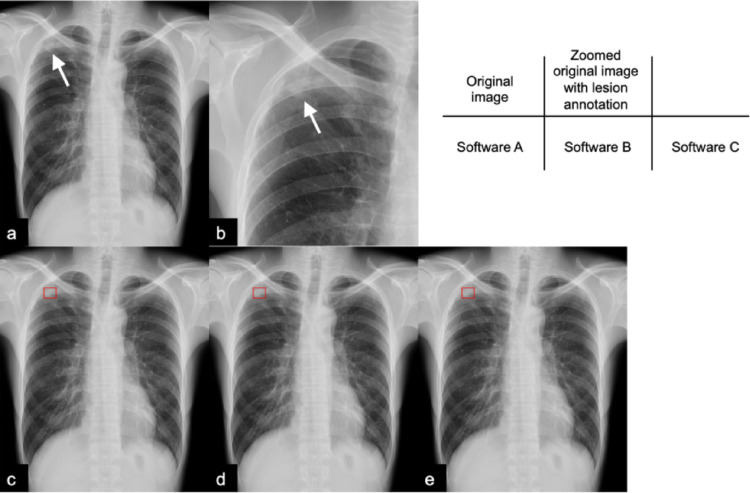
Representative case of concordant detection by first reader and all three AI model (46-year-old, male, BI = 260).  a and b. Chest radiograph showing a nodule in the right upper lung (arrow). c–e. All three AI models (Software A–C) correctly localized the same lesion, as indicated by red bounding boxes. The lesion’s visibility score was 2 (subtle)
